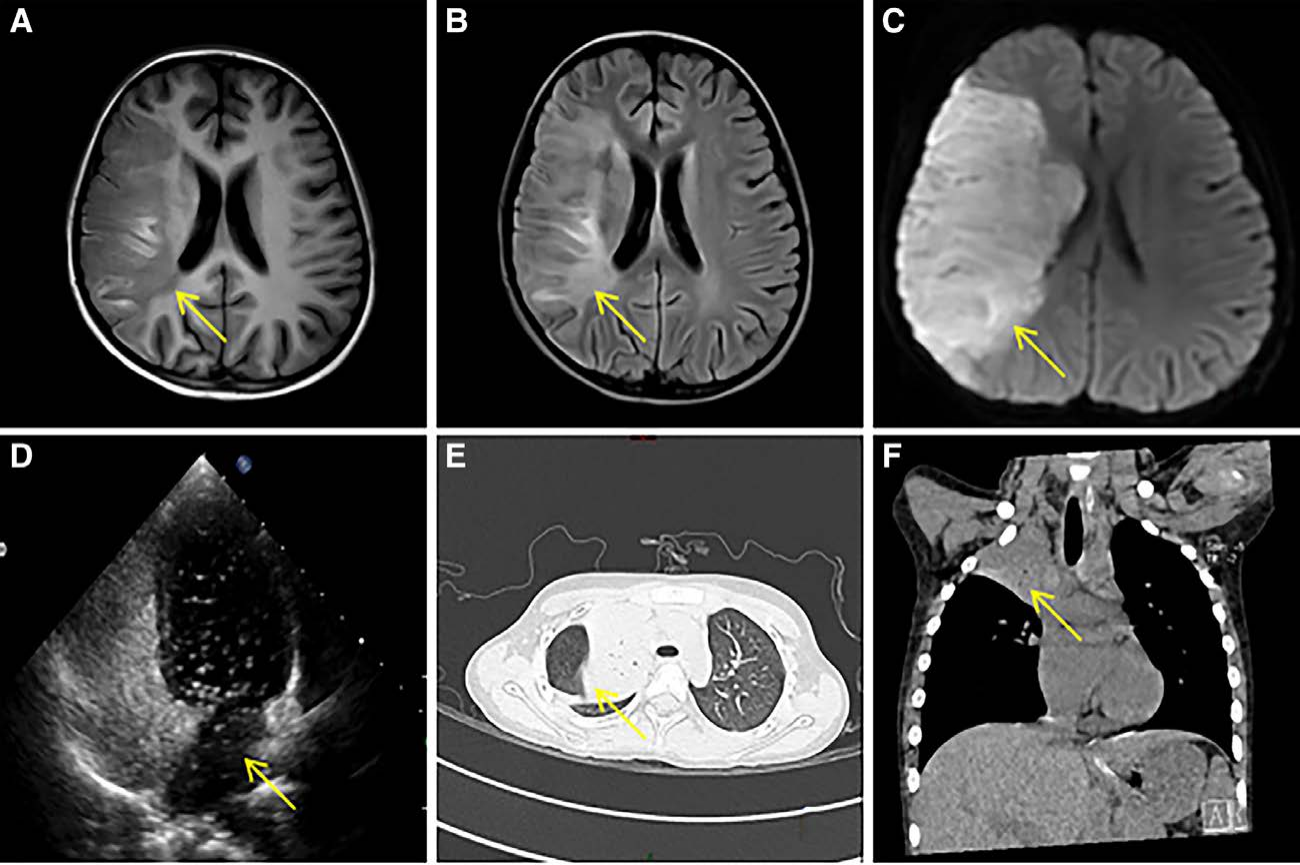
Examination results of case 2. (A) Cranial MRI: T1 sequence, ischemic stroke is a low-signal shadow; (B) Cranial MRI: T2 Flair sequence, ischemic stroke is a white high-signal shadow (yellow arrows); (C) Cranial MRI: Diffusion-weighted imaging sequence, ischemic stroke is a white high-signal shadow (yellow arrows), which is a typical manifestation of acute ischemic stroke; (D) The acoustic image of the right heart shows a large number of microbubbles in the left heart (yellow arrows); (E, F) The computed tomography of lungs: a large solid image of the right lung can be seen (yellow arrows). MRI = magnetic resonance imaging.
